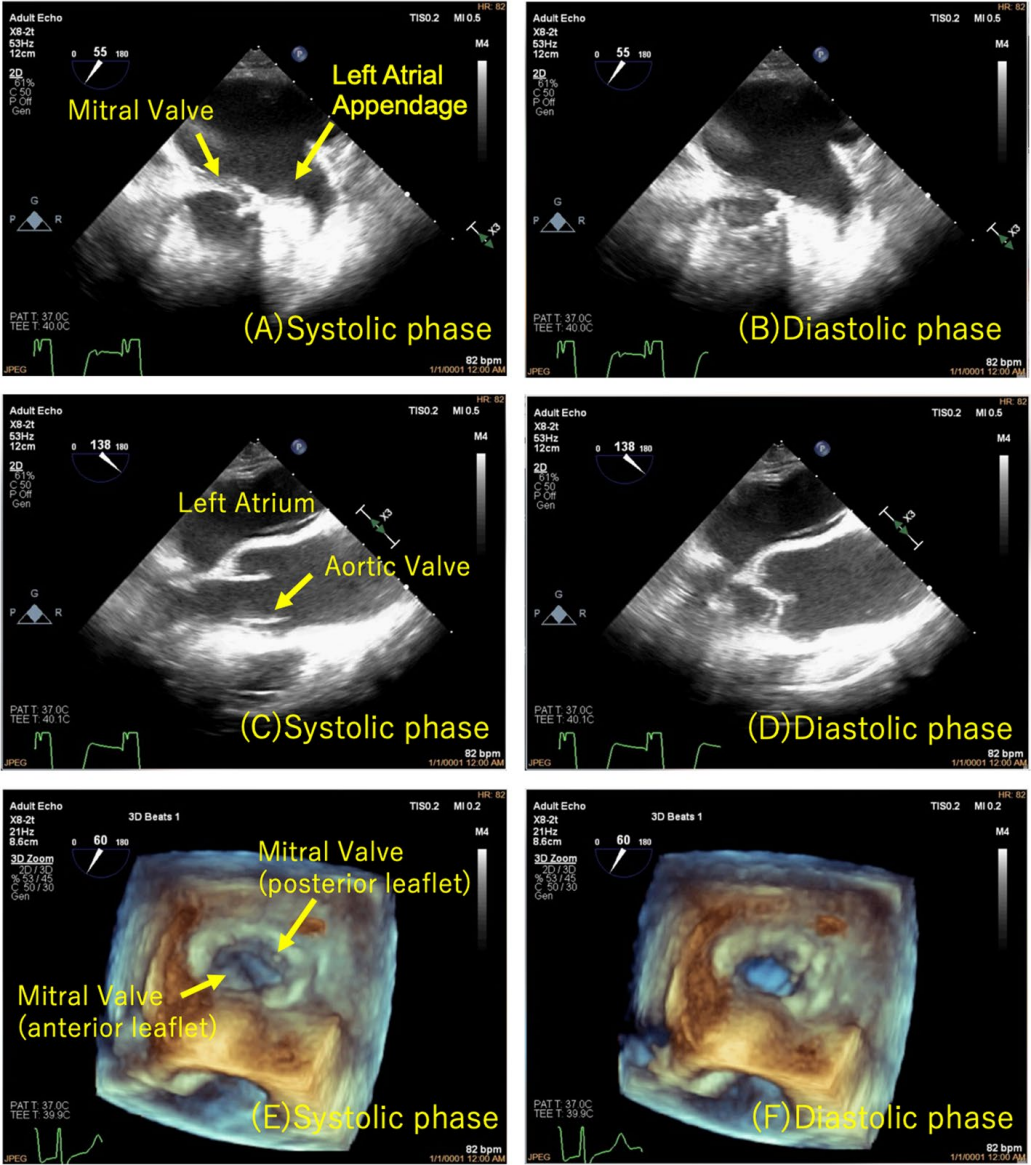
Transesophageal echocardiography during withdrawal from cardiopulmonary bypass (A mid-esophageal two-chamber image (systolic phase), B mid-esophageal two-chamber image (diastolic phase), C mid-esophageal long-axis image (systolic phase), D mid-esophageal long-axis image (diastolic phase), E 3-dimensional TEE (systolic phase), F 3-dimensional TEE (diastolic phase)). During withdrawal from cardiopulmonary bypass, image quality and field of view were improved, and the mitral valve could be observed. No thrombus or large air volume was observed in the left atrium (A–B), and the mitral valve was deemed sufficiently repaired (A–F)
